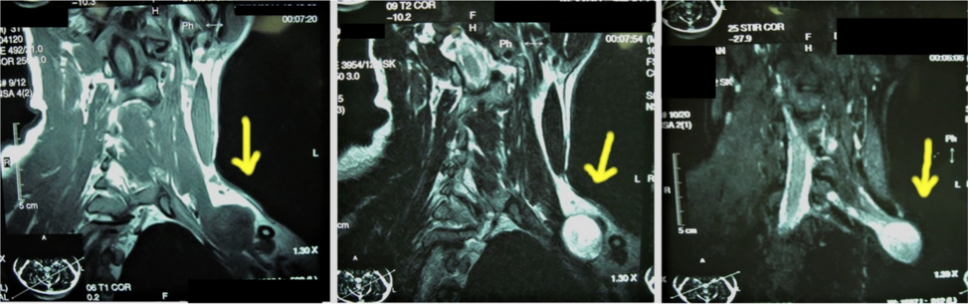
MRI neck showing well circumscribed lesion involving C7 root with enhancement on T2 weighted images.
